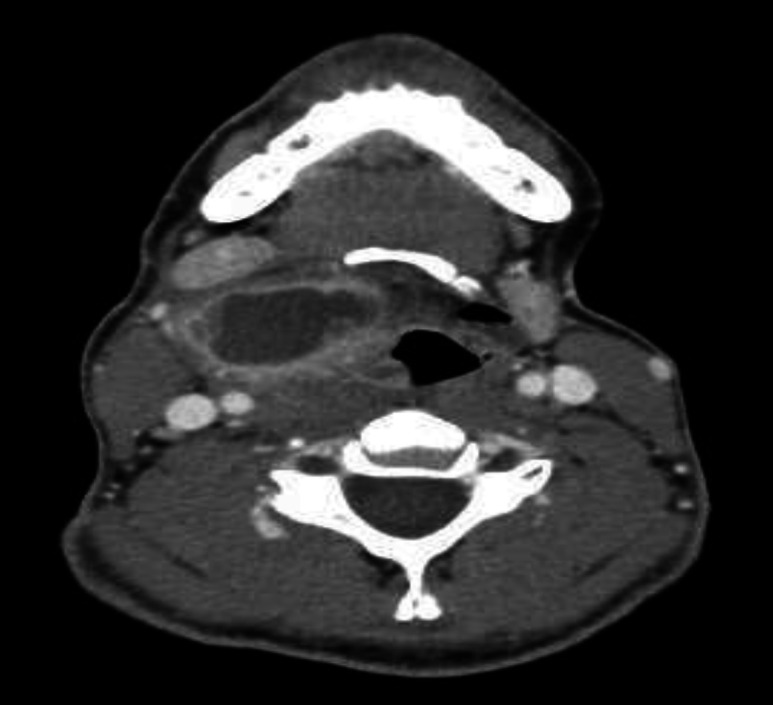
Axial CT scan of the neck during previous hospitalization
